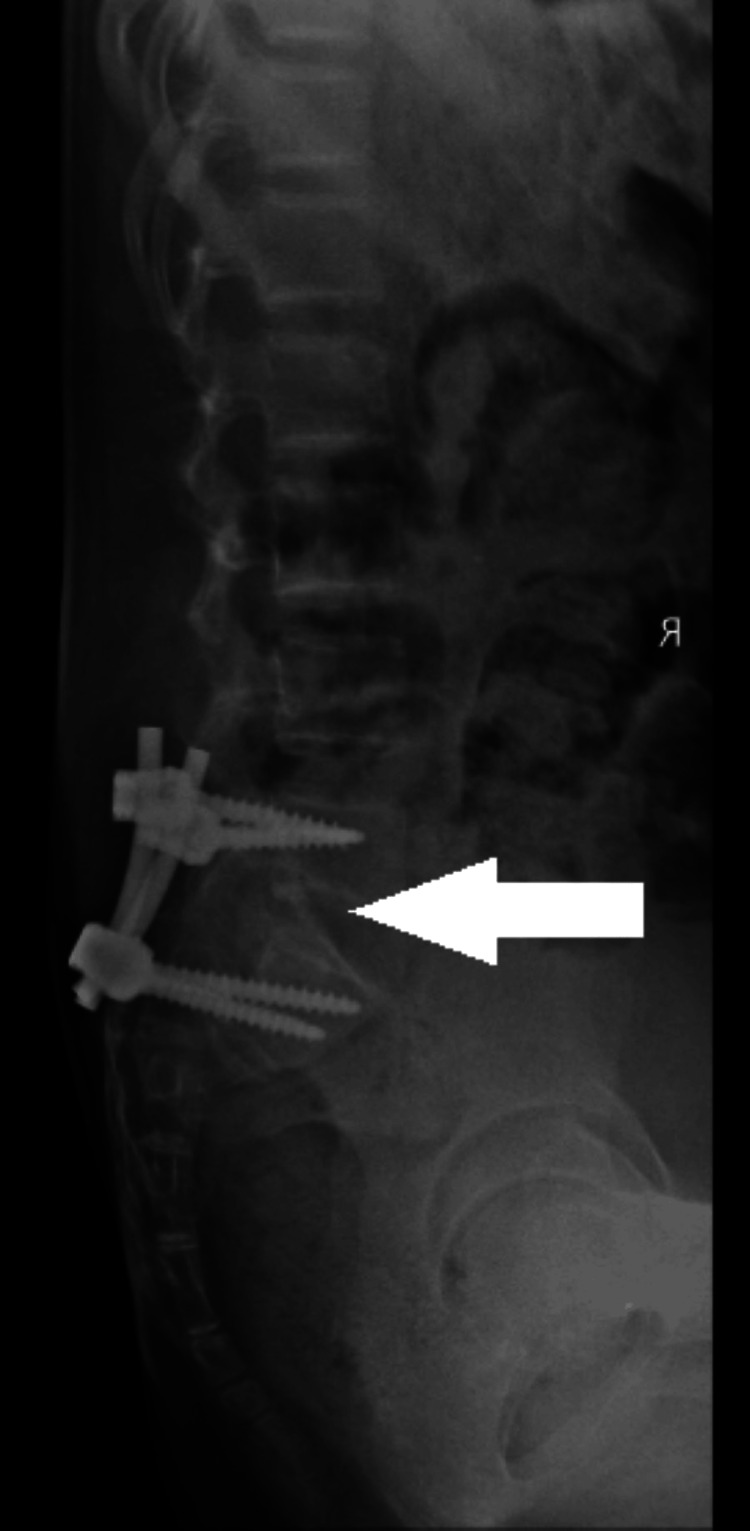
Lateral X-ray of the lumbar spine at the eighth-month follow-up demonstrating evidence of fusion (white arrow)
